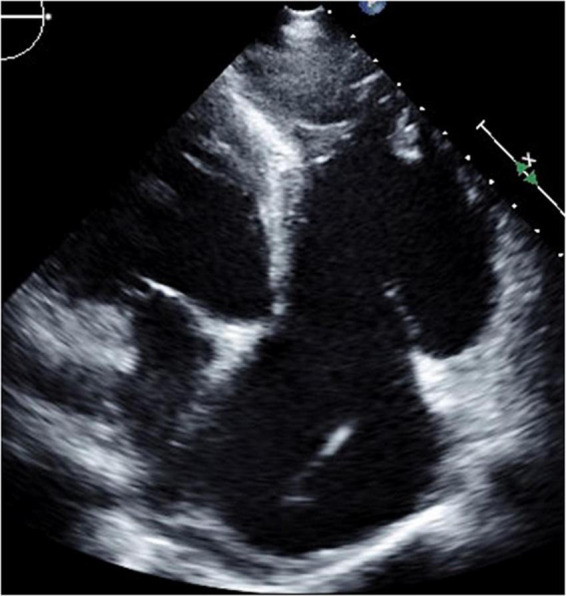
Four-chamber view at end-systolic phase of transthoracic echocardiography in Case 4. Note that the tricuspid valve does not close at all, and the right atrium is hugely dilated.
